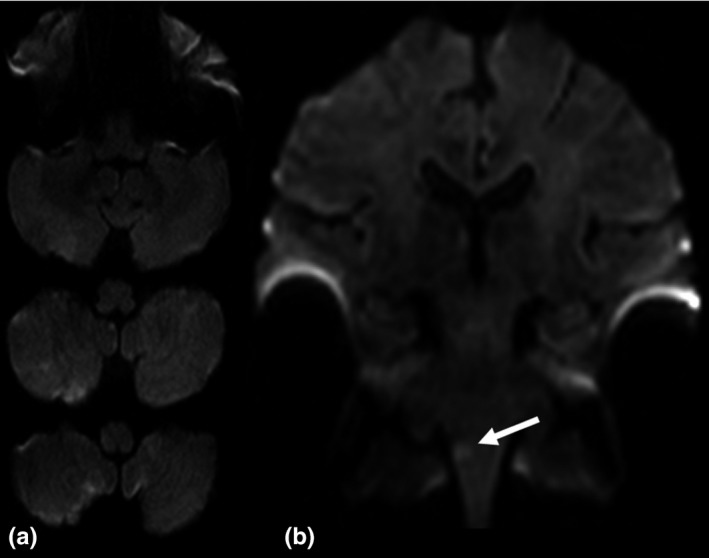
Acute ischemic infarction in the right medulla oblongata. (a) On standard axial diffusion‐weighted imaging (DWI) the ischemic lesion is not visible. (b) On thin‐section coronal DWI the ischemic lesion is clearly delineated (arrow)
